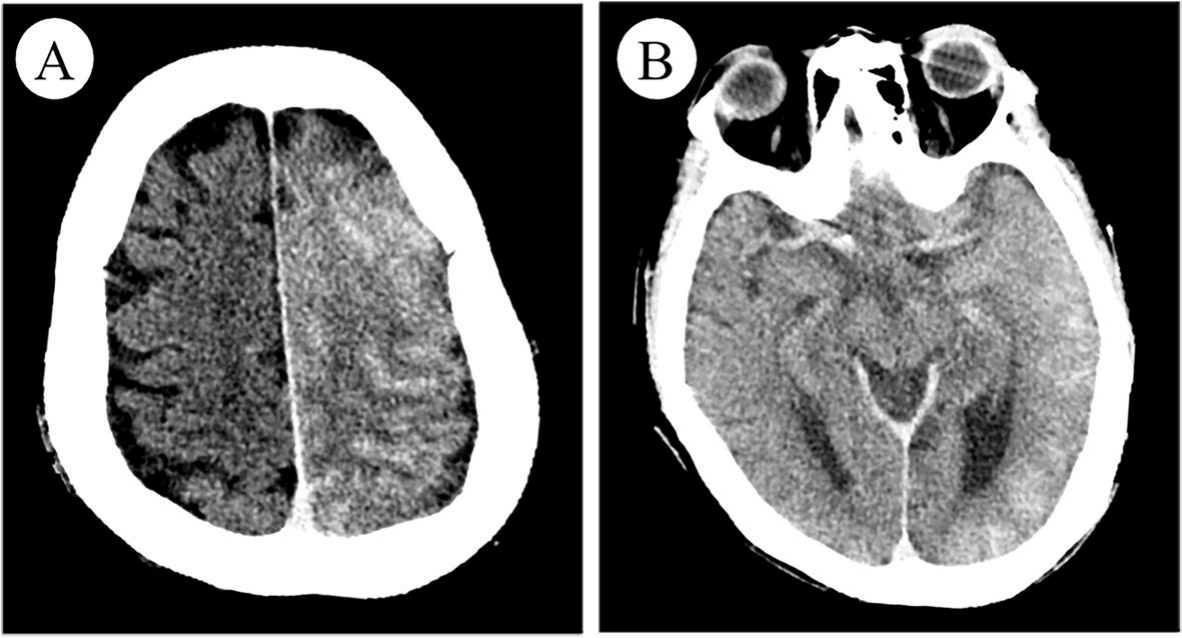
Emergent brain CT angiography (CTA). Axial brain CTA showing the left cerebral hemisphere swelling and diffuse high-density in the subarachnoid space
